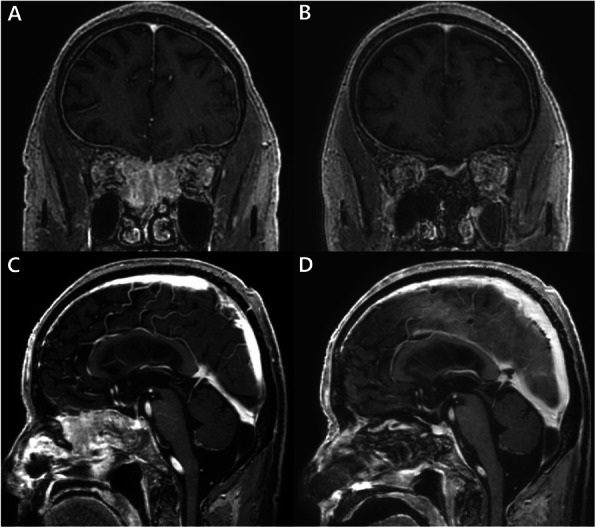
a, c A 78-year old male patient with huge olfactory neuroblastoma with extensive ASB defect which was revealed in preoperative enhanced coronal and sagittal MRI. b, d Postoperative coronal and sagittal MRI showed insufficient anterior coverage of PNSF for ASB defect, and loose attachment of PNSF to the skull base despite the support of tissue glue and nasal packing. The patient encountered the CSF rhinorrhea 3 days after the operation. ASB: anterior skull base, PNSF: pedicled nasoseptal flap
